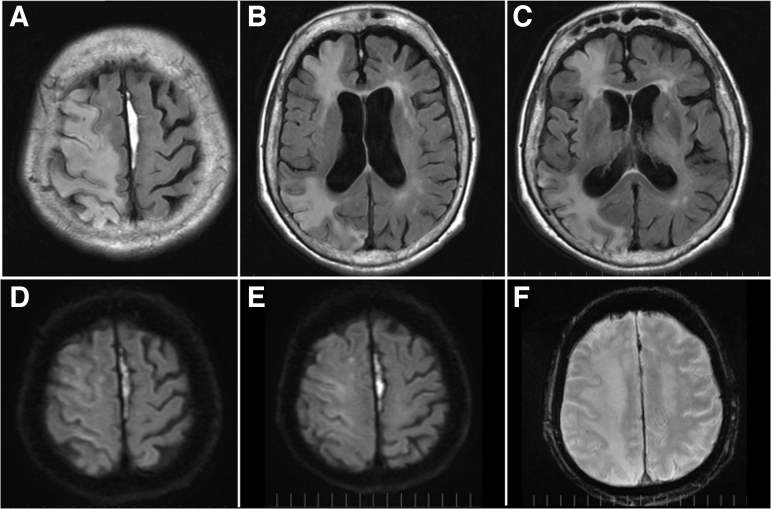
Magnetic resonance imaging on day 34. (A–C) Fluid-attenuated inversion recovery imaging showed a patchy high signal in the right cerebral hemisphere. (D,E) Diffusion-weighted images showed high signal in the right frontal cortex and center of the semicircle. (F) T2* (star) -weighted image showed a punctate low signal in the subcortical area of the right parietal lobe, suggestive of an old microhemorrhage.
